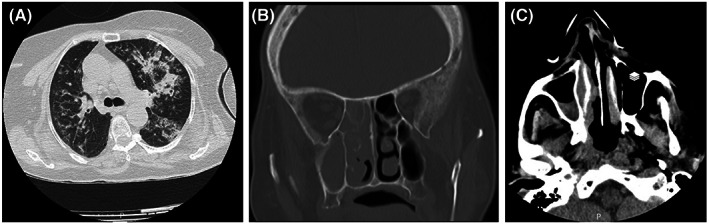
(A) Chest CT scan demonstrating multiple peripheral patchy grand glass and consolidation with air bronchogram, highly suggestive of COVID‐19 pneumonia. (B and C) Paranasal CT scan showing mucosal thickening and opacification in right maxilla, frontal and ethmoid sinuses, and right and left sphenoids.
